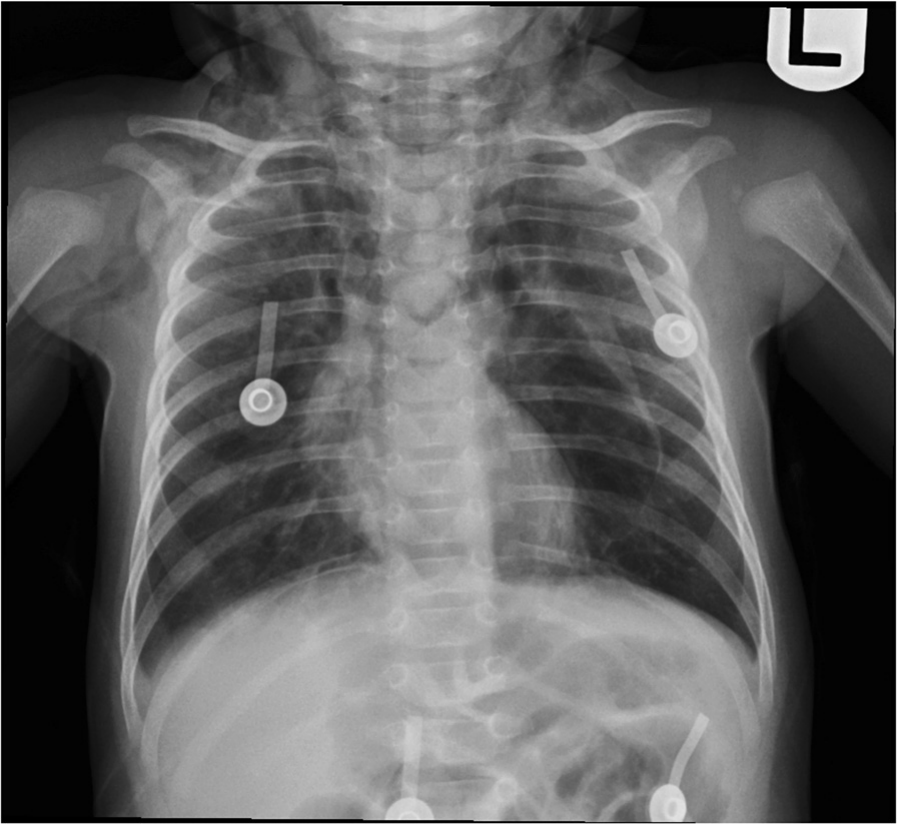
Chest X-ray. Evidence of the pneumomediastinum and soft tissue emphysema extends to the root of the neck. The lungs demonstrate bilateral heterogeneous opacities
